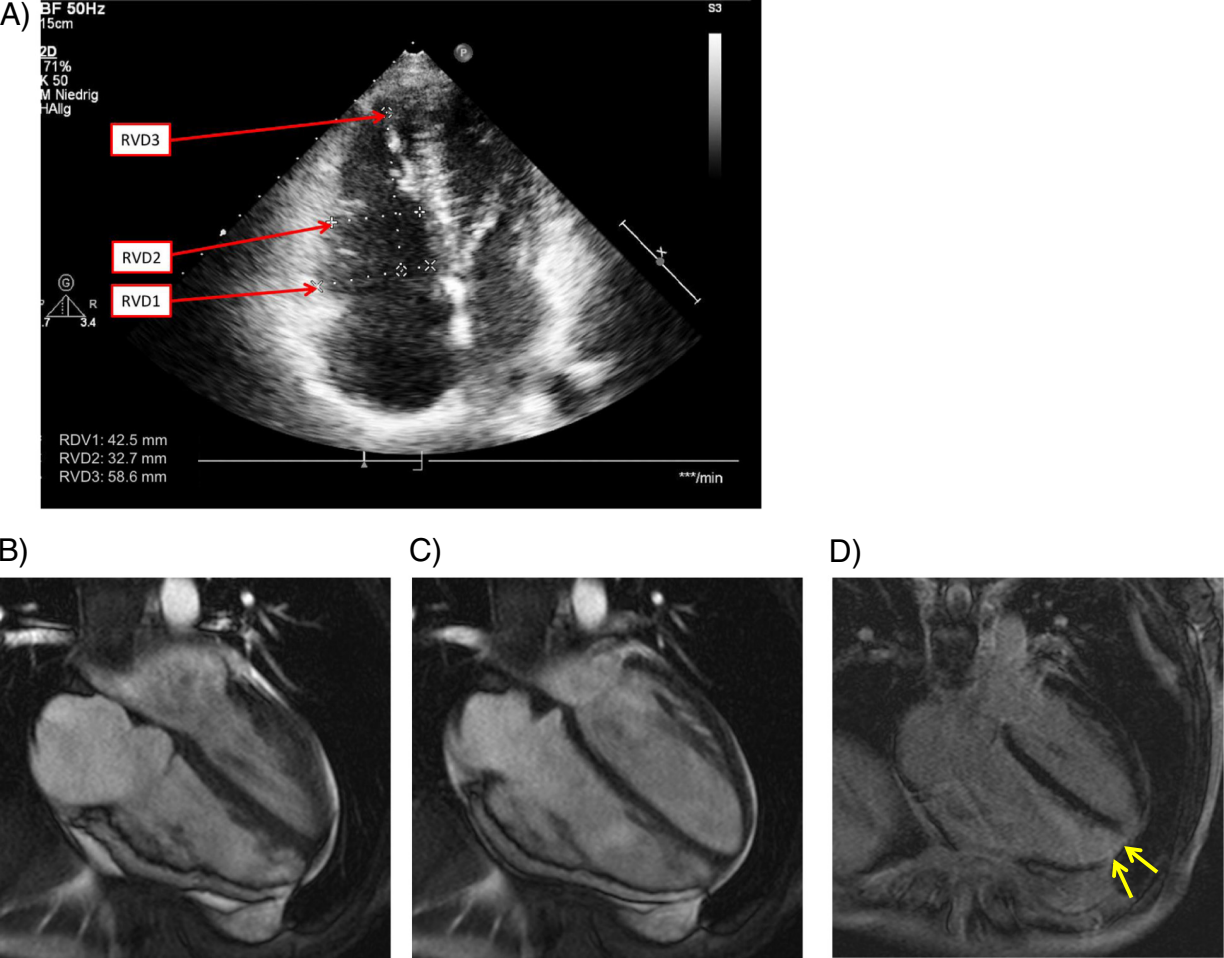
a Echocardiography of index patient shows dilatation of the right ventricle. RVD1 (basal right ventricle): 42.5 mm (Mean value (95 % CI) 33 (31–35 mm)). RVD2 (mid right ventricle) 32.7 mm (Mean value (95 % CI) 28 (23–33 mm)). RVD3 (base to apex length) 58.6 mm (Mean value (95 % CI) 71 (67–75 mm)). Mean values (95 % CI) according to Rudski et al. [9]. b Cardiac MRI of the index patient reveals moderate enlargement of the right ventricle with an enddiastolic volume of 186 ml (standard value female 140 ± 37 ml) and (c) endsystolic volume of 135 ml (standard value female 52 ± 22 ml) resulting in a highly reduced ejection fraction of 27 % (standard value female 64 ± 7 %) Furthermore, the apical posterior wall of the right ventricle showed akinesia and (d) late gadolinium enhancement identified apical fibrosis (yellow arrows) in the 4-chamber view. Standard values according to Hudsmith et al. [10]
